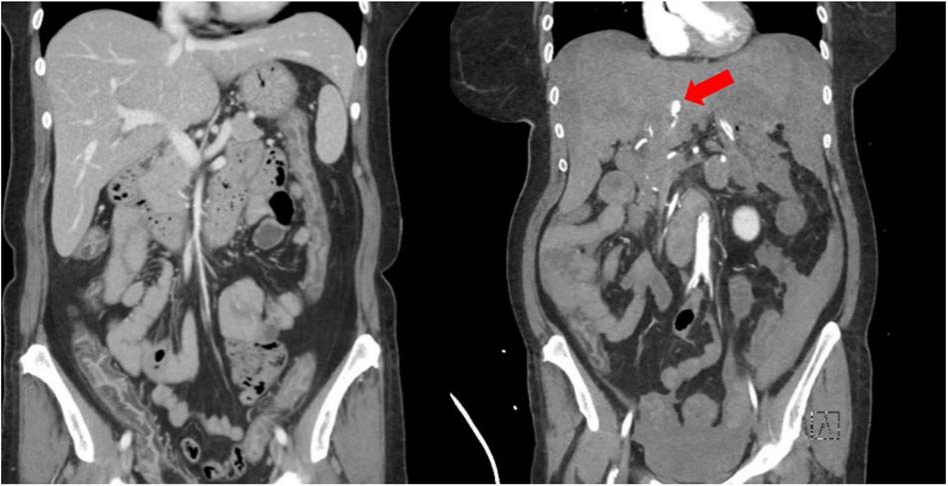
Imaging showing initial CT scan (left) and repeat CT angiogram (right) several hours later. Red arrow is identifying left HAP.
